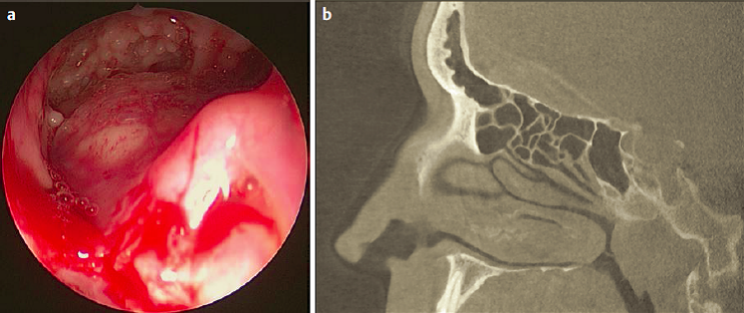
Exostoses of the frontal sinus, endoscopic view (a) and CT scan (b)
